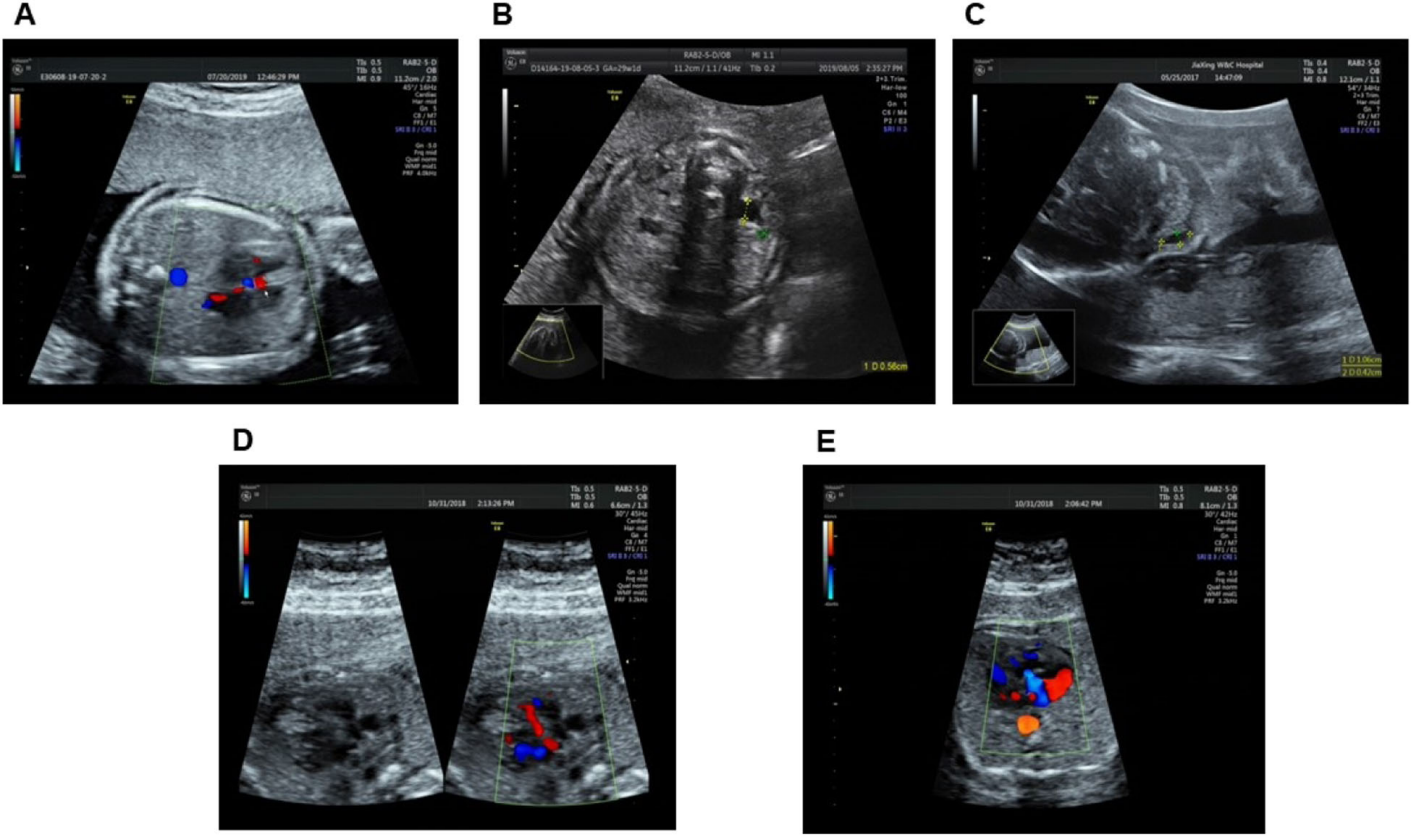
Prenatal ultrasonographic findings of the fetus. a P1 fetus at 23 weeks gestational age, showing ultrasonographic findings of tetralogy of Fallot. b P1 fetus, showing ultrasonographic findings of left kidney collection system separation. c P2 fetus, showing ultrasonographic findings of a water cyst in the neck. d P3 fetus, showing ultrasonic findings of tricuspid regurgitation. e P3 fetus, showing a perimembranous ventricular septal defect
